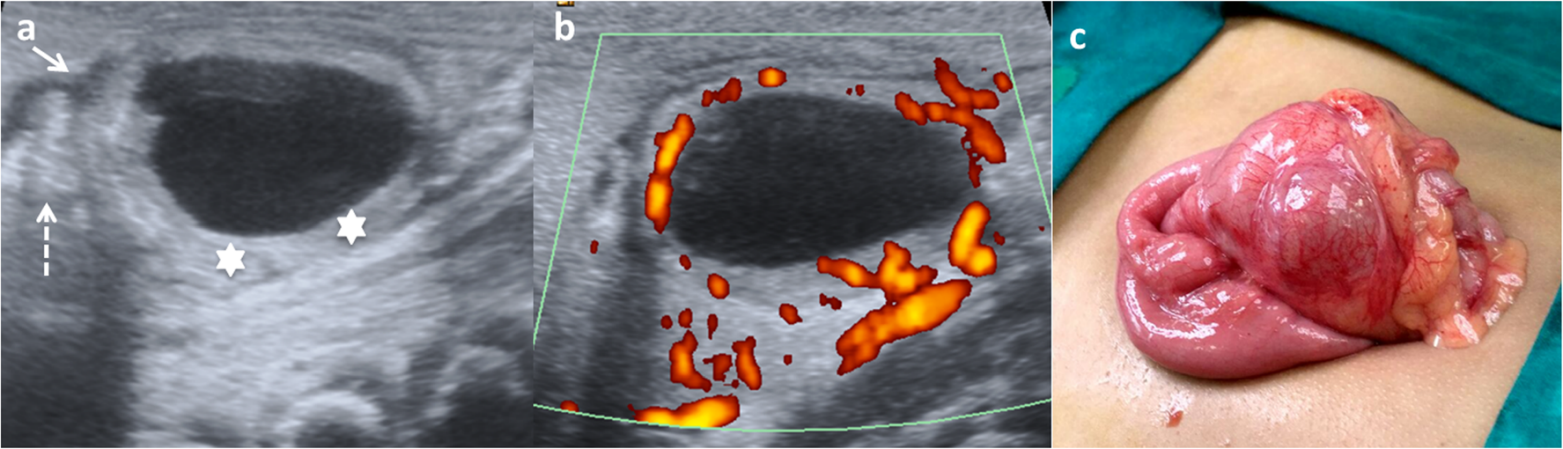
A 3-week-old term newborn with abdominal distention and gastric intolerance. a Longitudinal US view of the right low quadrant: thickened wall (*stars*) cystic lesion next to a bowel loop (*dashed-line arrow*). The “Y” sign between the bowel and the lesion (*arrow*). b Power Doppler US demonstrates the significant vascularisation in the cyst wall. c Surgical findings: a cystic tumour next to the ileocecal valve. Pathological findings: ileal duplication cyst with heterotopic pancreatic tissue
